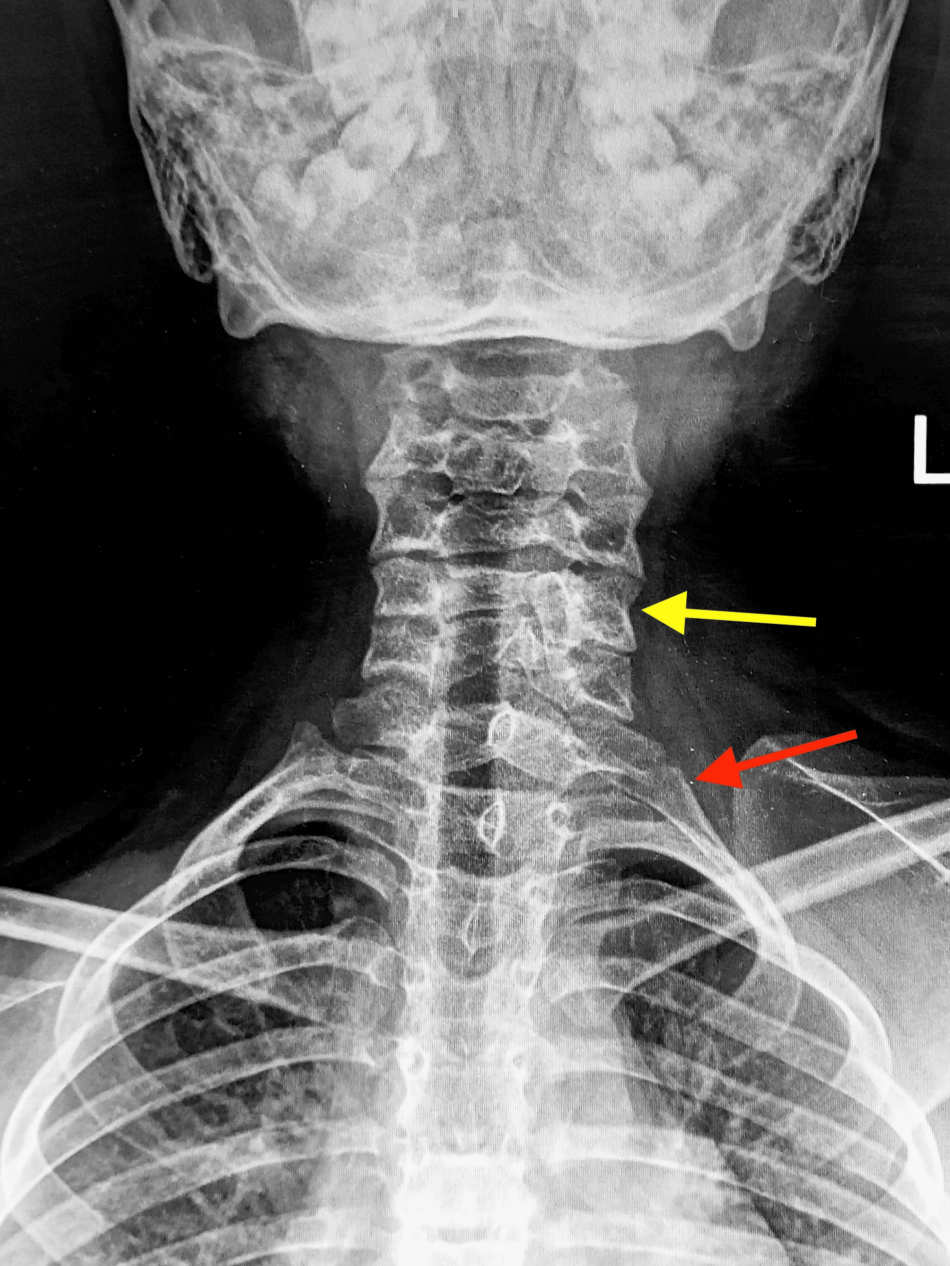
An anteroposterior radiograph of the cervical spine showing spina bifida of the C5 vertebrae (yellow arrow) and left cervical rib (red arrow)
